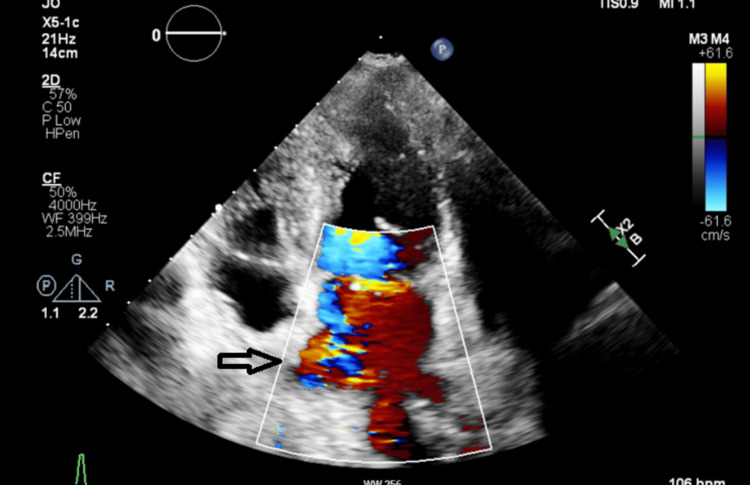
Transthoracic echocardiography: severe mitral valve regurgitation.
